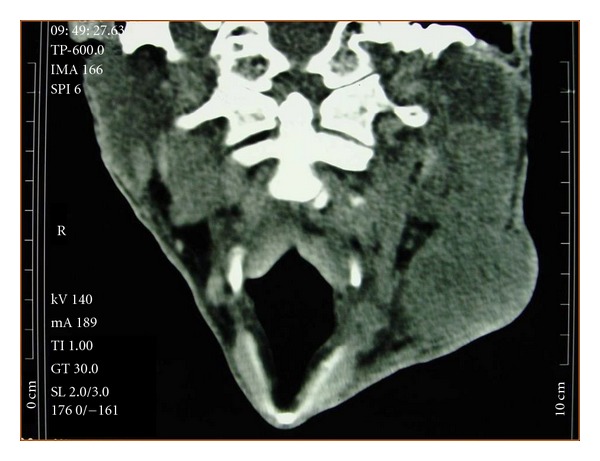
Computed tomography scan showing the lymph nodes metastases of Merkel cell carcinoma.
